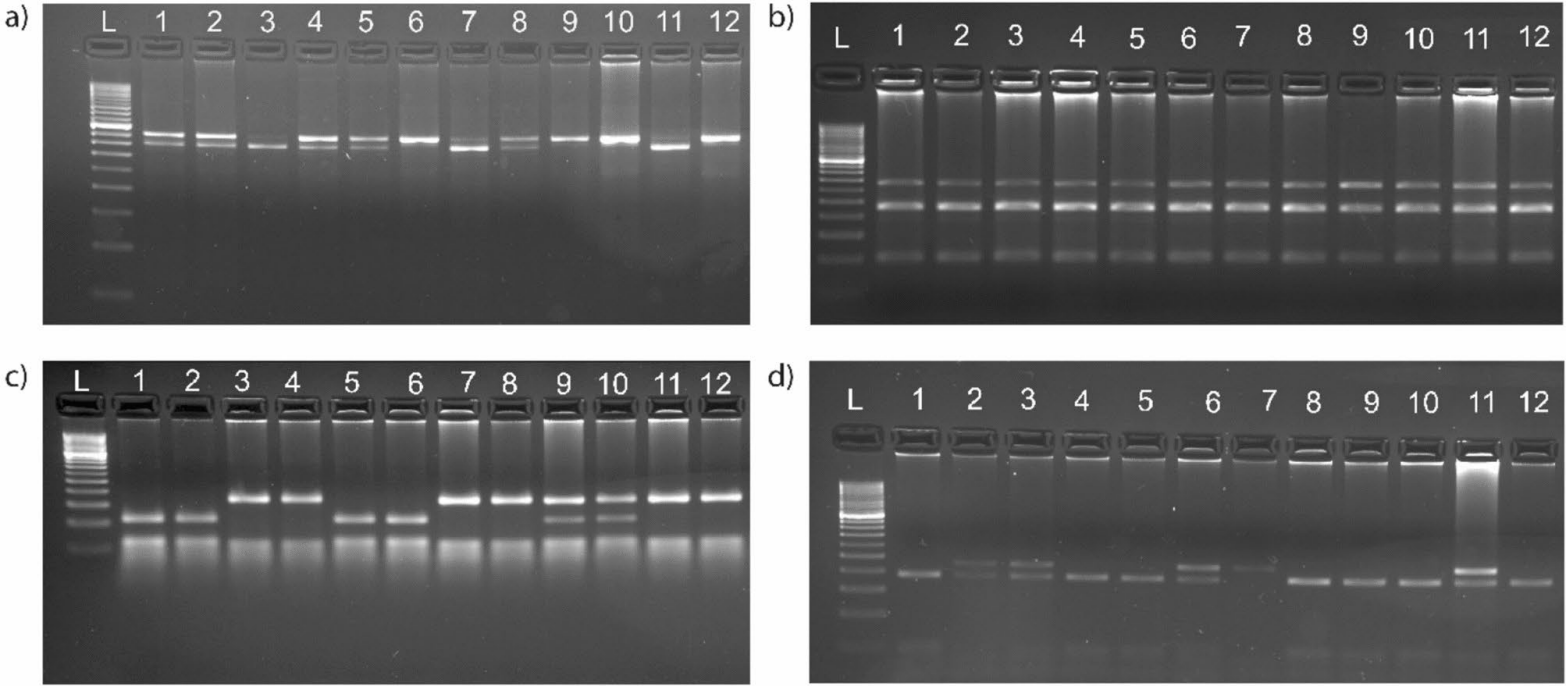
Representative image of PCR–RFLP: (a) *Notch1*; lane 3 and 11: CC genotype (397 and 43 bp) lane 6, 9, 10, and 12 TT genotype (440 bp) and lane 1, 2, 4, 5, and 6: TC genotype (440, 397, and 43 bp) (b) *Notch2*; lane 1–12 showing TT genotype (228 and 100 bp) (c) *Notch3*; lane 1,2,5, and 6 GG genotype (107 and 61 bp), lane 3,4,7,8,11, and 12 AA genotype (168 and 61 bp), lane 9 and 10: GA genotype (168 bp, 107 bp, and 61 bp) (d) *Notch4*; lane 1,4,5,8,9,10, and 12: CC genotype (190 and 40 bp), lane 2,3, and 6 CT genotype (230, 190, and 40 bp) and lane 7: TT genotype (230 bp) in different samples. L-50 bp DNA ladder.
